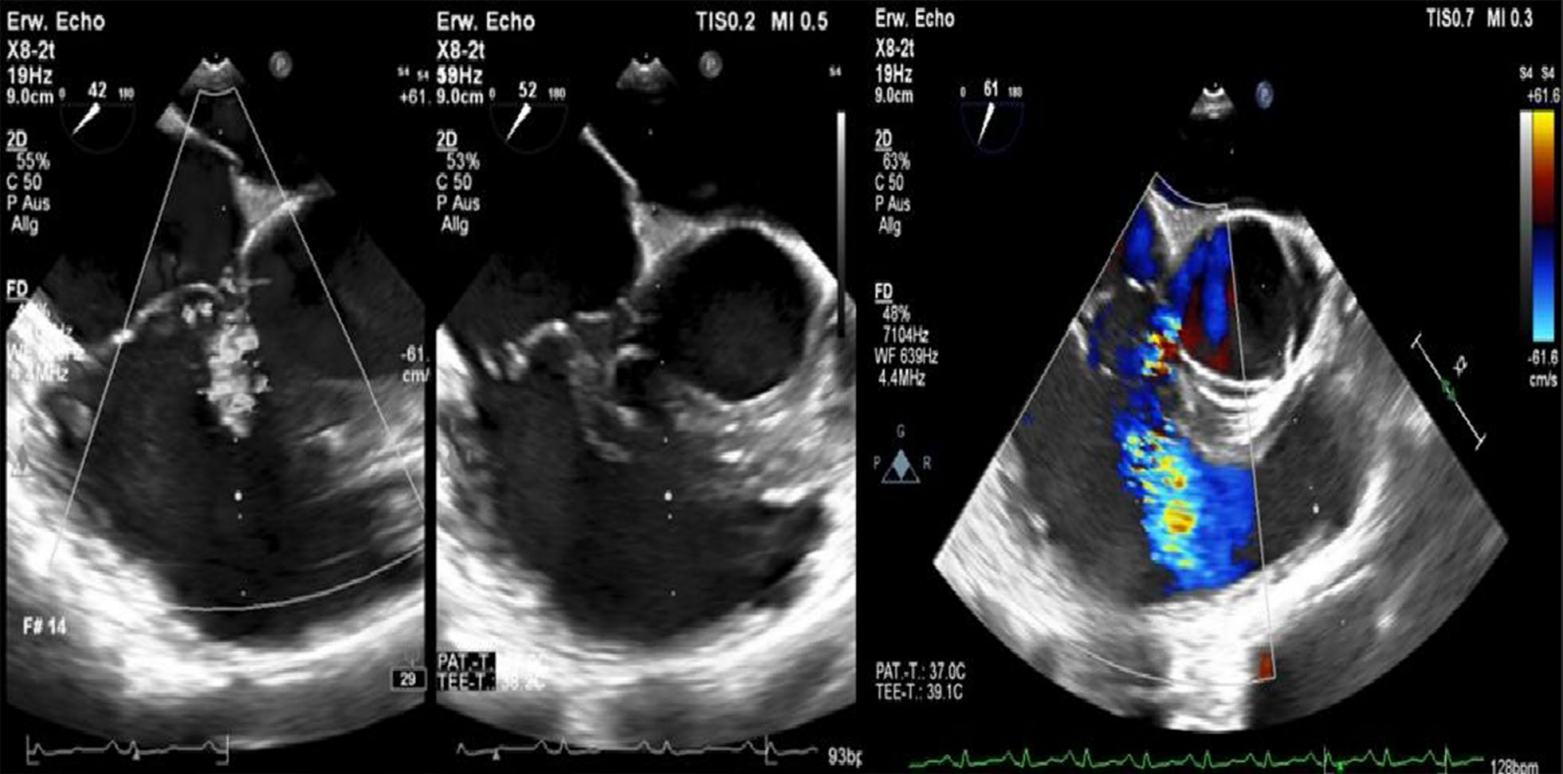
Transoesophageal echocardiography showing a VSD corresponding to a type 2 Gerbode defect
